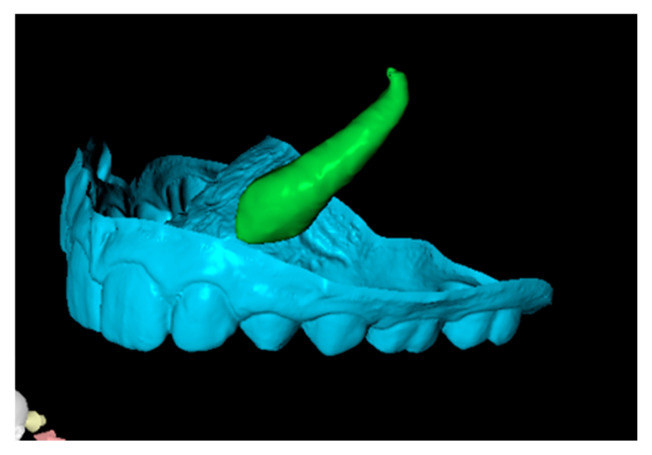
Initial STL with the IC from the CBCT scan.
